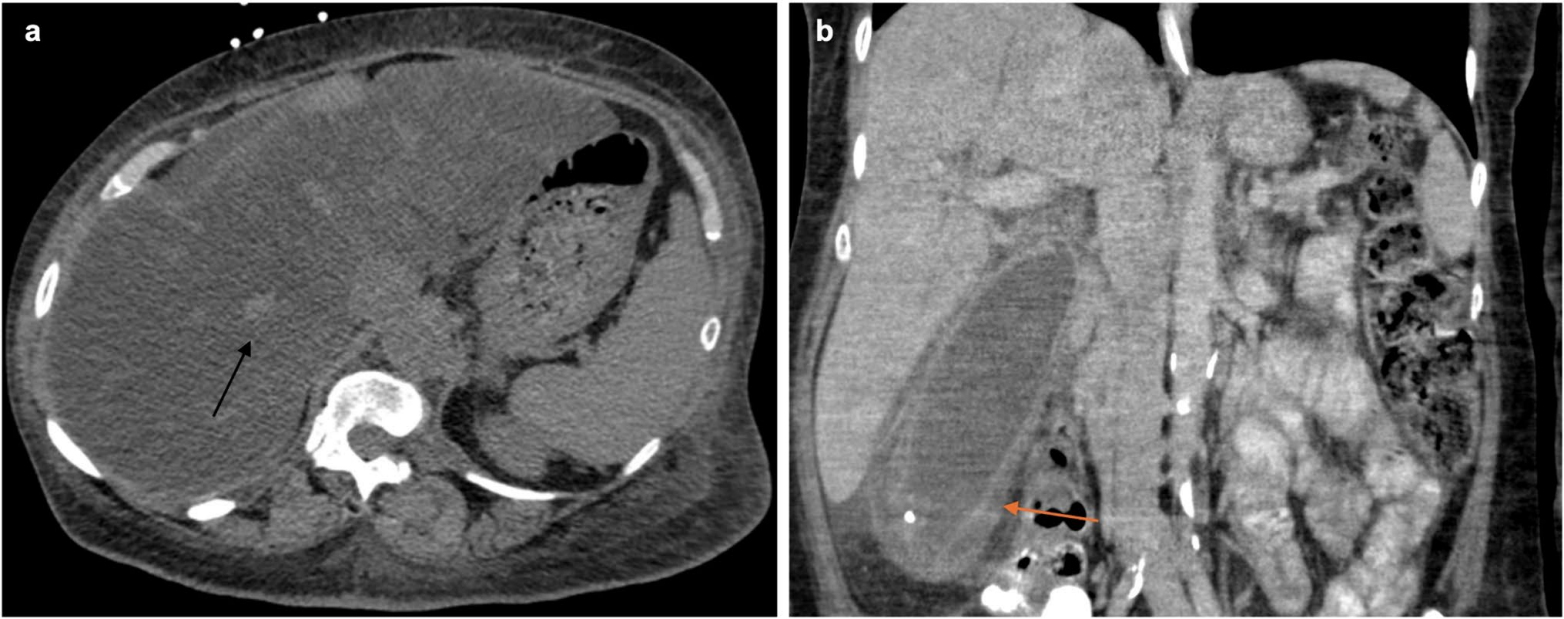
Findings associated with toxicity from usage of checkpoint inhibitors. CT abdomen (a) demonstrating hepatitis (black arrow) with hepatomegaly, heterogeneous hepatic contrast enhancement, well-defined parenchymal zones with low attenuation, and periportal hypoattenuation. Coronal CT (b) demonstrates gallbladder distention, mucosal hyperemia, wall thickening, pericholecystic fat stranding/fluid consistent with cholecystitis (orange arrow)
